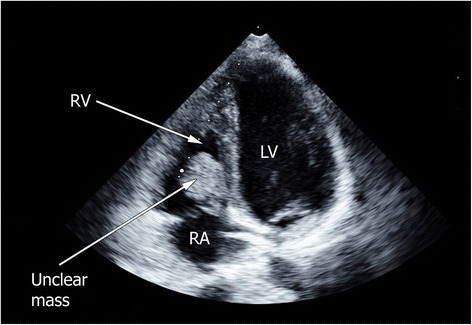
Right ventricular mass. Echocardiographic image of the thrombus in the right ventricle. *LV* Left ventricle, *RA* Right atrium, *RV* Right ventricle
